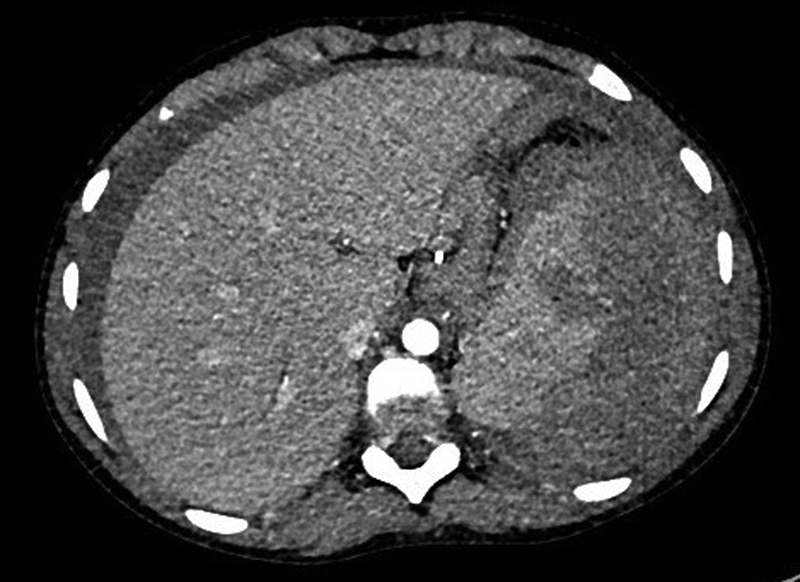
Axial image from the contrast-enhanced computed tomography scan performed on admission, showing rupture of the lateral margin of the spleen and a large perisplenic hematoma. Free fluid is also seen lateral to the liver.
